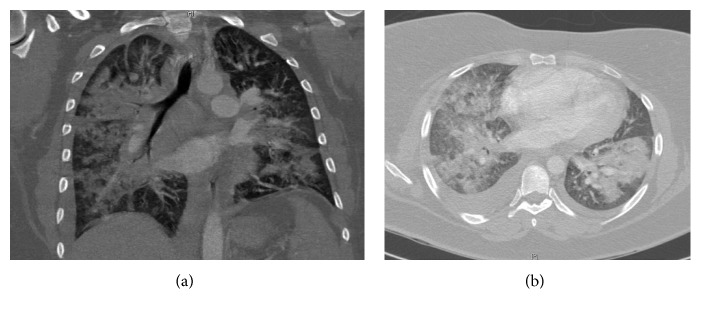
Radiographic appearance at presentation.
